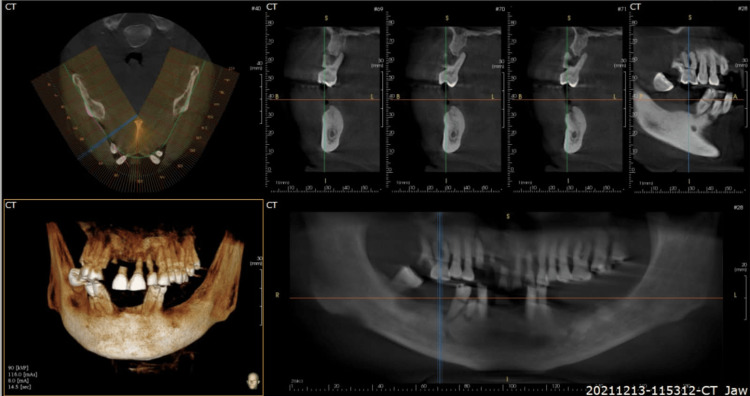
Pre-operative cone-beam computed tomography (Case 2)
